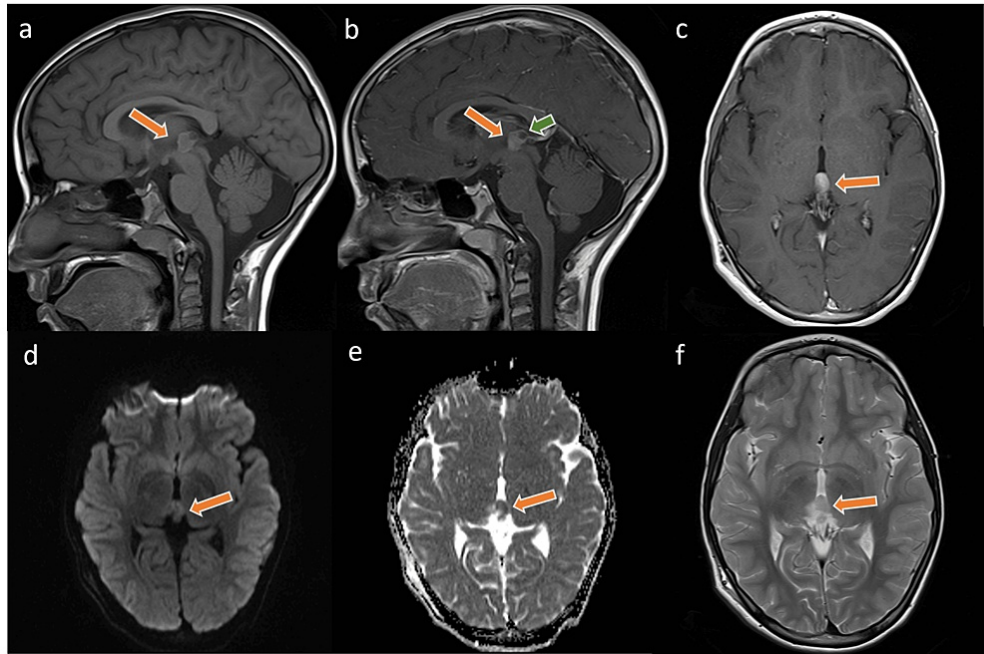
MRI images at initial diagnosis Few selected MR images of the brain at the time of the initial diagnosis demonstrate the well-defined pineal lesion (arrow). The lesion is of an intermediate signal in the T1-weighted images with a rim of high signal (a) and low T2-weighted images signal intensity (c) with enhancement on the post-contrast images as seen in the sagittal (b) and axial (f) planes. Noted is the diffusion restriction signal in the DWI sequences (d, e). Intralesional cystic (green arrow) changes are identified and no evidence of tectal infiltration was found.
